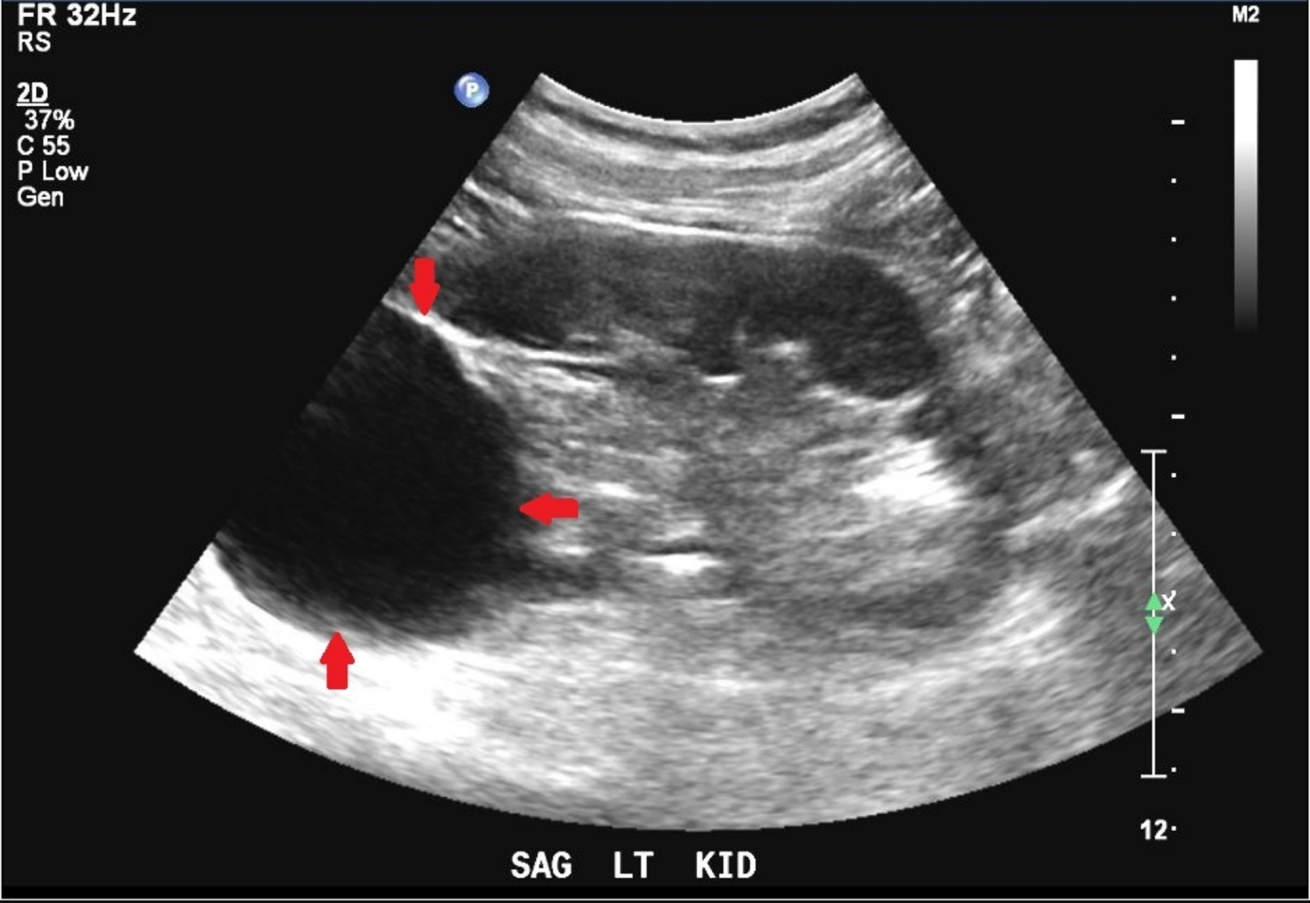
Abdominal ultrasound showing a 6.2 cm cyst at the upper pole of the left kidney.
